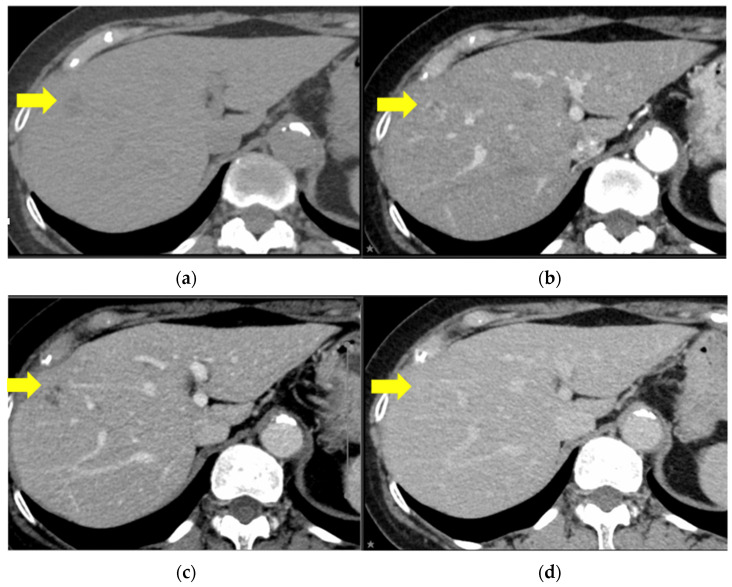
Contrast enhanced computed tomography images. There is a round 27 × 26 mm lesion in S8 with mild ring-enhancement during the arterial phase and an irregularly contrasted hypodense area within the lesion. The yellow arrows indicate the features listed. (a) Plain, (b) arterial phase, (c) portal phase, and (d) equilibrium phase.
